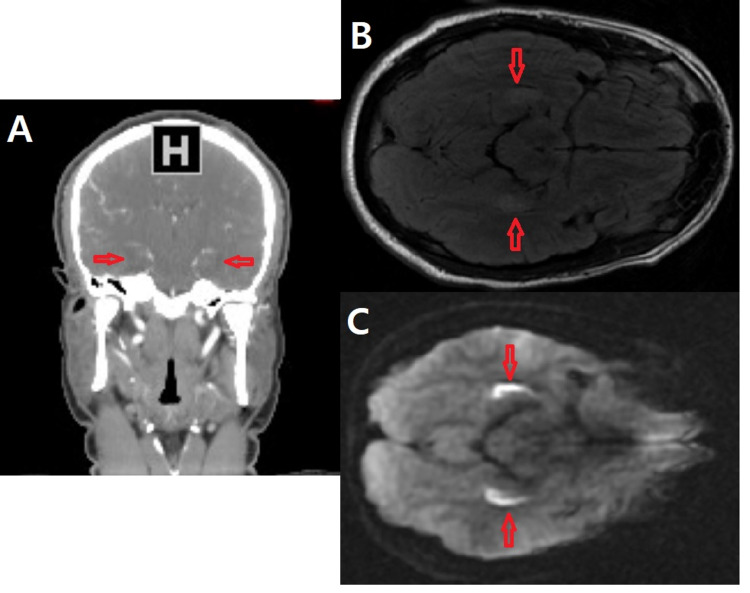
Cerebral imaging studies of a patient presenting with dense anterograde amnesia, with short interval retrograde amnesia, and concomitant IV fentanyl use. A. CTA (coronal view) demonstrates relative hypoattenuation along the bilateral hippocampi B. MRI FLAIR (axial view) demonstrates high signal intensity along the bilateral hippocampi C. Diffusion-weighted MRI (axial view) demonstrates high signal along the bilateral hippocampi correlating with restricted diffusion (ADC image not shown) CTA, CT angiography; FLAIR, fluid-attenuated inversion recovery
